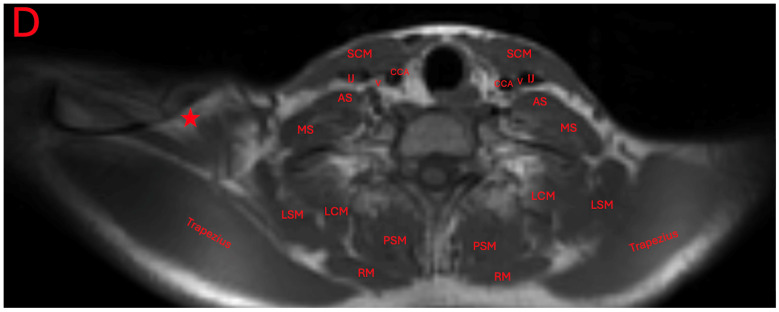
Axial T1-weighted MRI imaging of the patient’s neck and shoulder (A–D). Red stars indicate the position of the levator claviculae muscle. The other abbreviations are the common carotid artery (CCA), vagus nerve (V), internal jugular vein (IJ), sternocleidomastoid (SCM), anterior scalene muscle (AS), middle scalene muscle (MS), omohyoid (OMO), levator scapulae muscle (LSM), longissimus cervices muscle (LCM), paraspinal muscle (PSM), and rhomboid muscle (RM).
